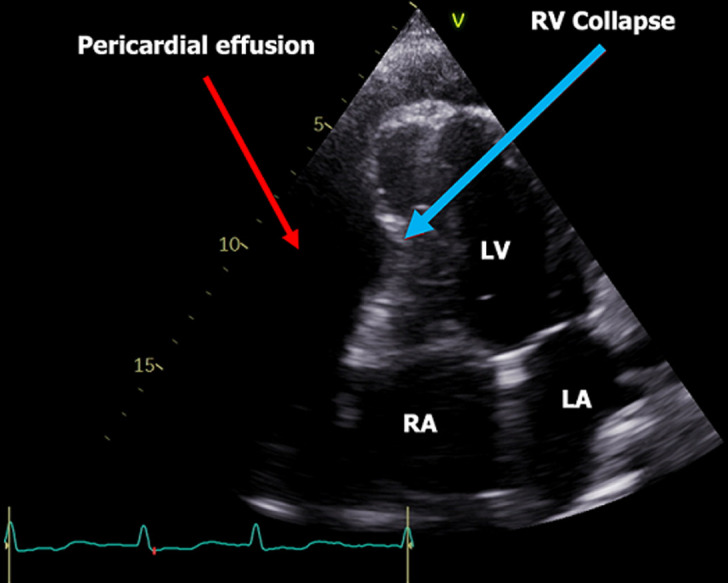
a two-dimensional transthoracic echocardiography reveals a significant pericardial effusion surrounding the heart; the red arrow indicates the effusion, while the blue arrow indicates right ventricular (RV) collapse; (LV: left ventricular, LA: left atrium, RA: right atrium)
